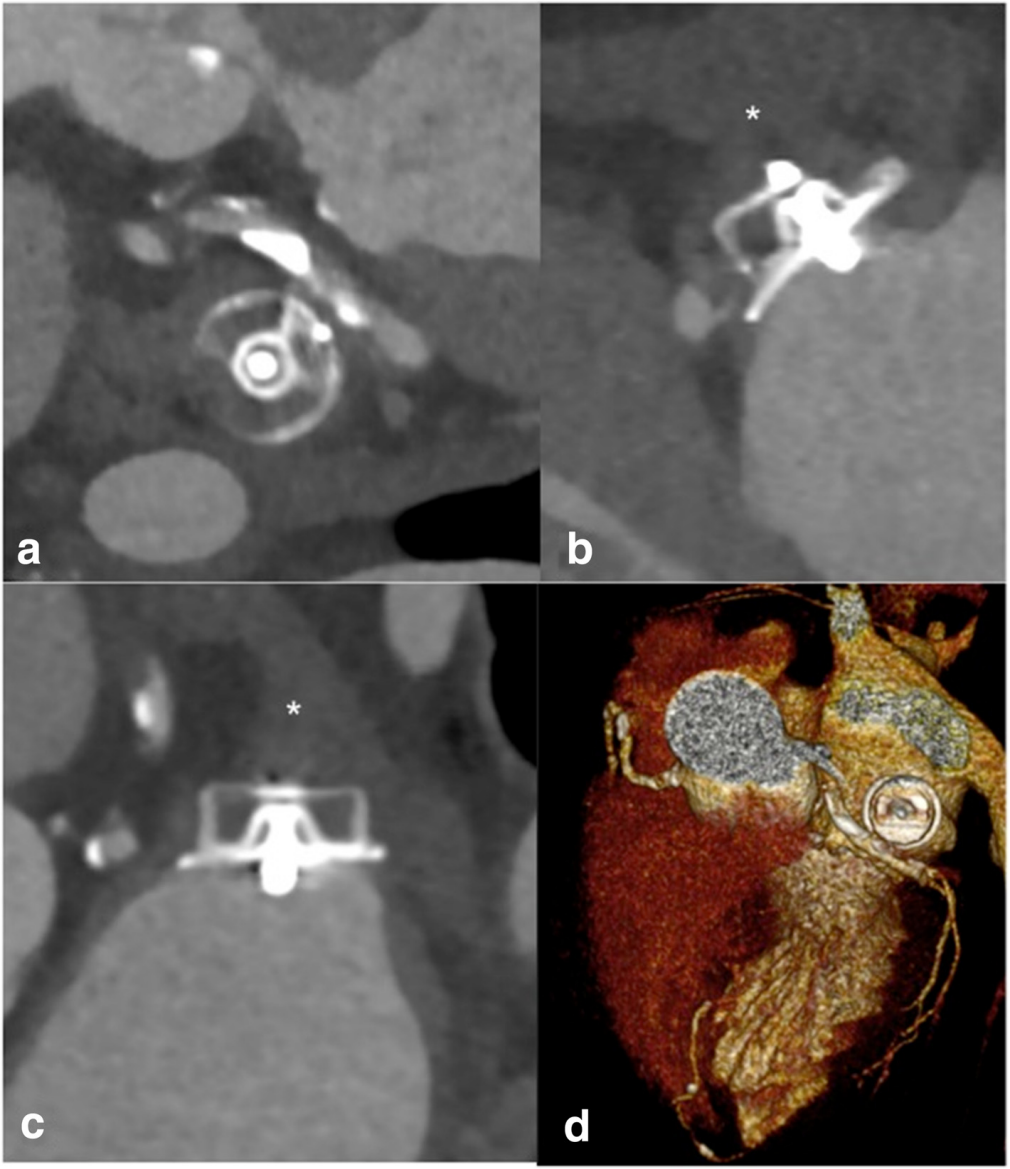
LOVE - axial (panel a), − sagittal (panel b), - coronal (panel c), and 3D (panel d) reformations of a 72 year-old male patient who underwent cardiac CTA 9 months after implantation of a ACP® LAA occlusion device. Panels a, b, and c show optimal positioning of the device with no peri-device leak, complete lobe coverage as well as complete neo-endothelialization of the device suggested by the absence of contrast enhancement < 50 Hounsfield units in the LAA (* in B and C). Volume rendered reconstructions
